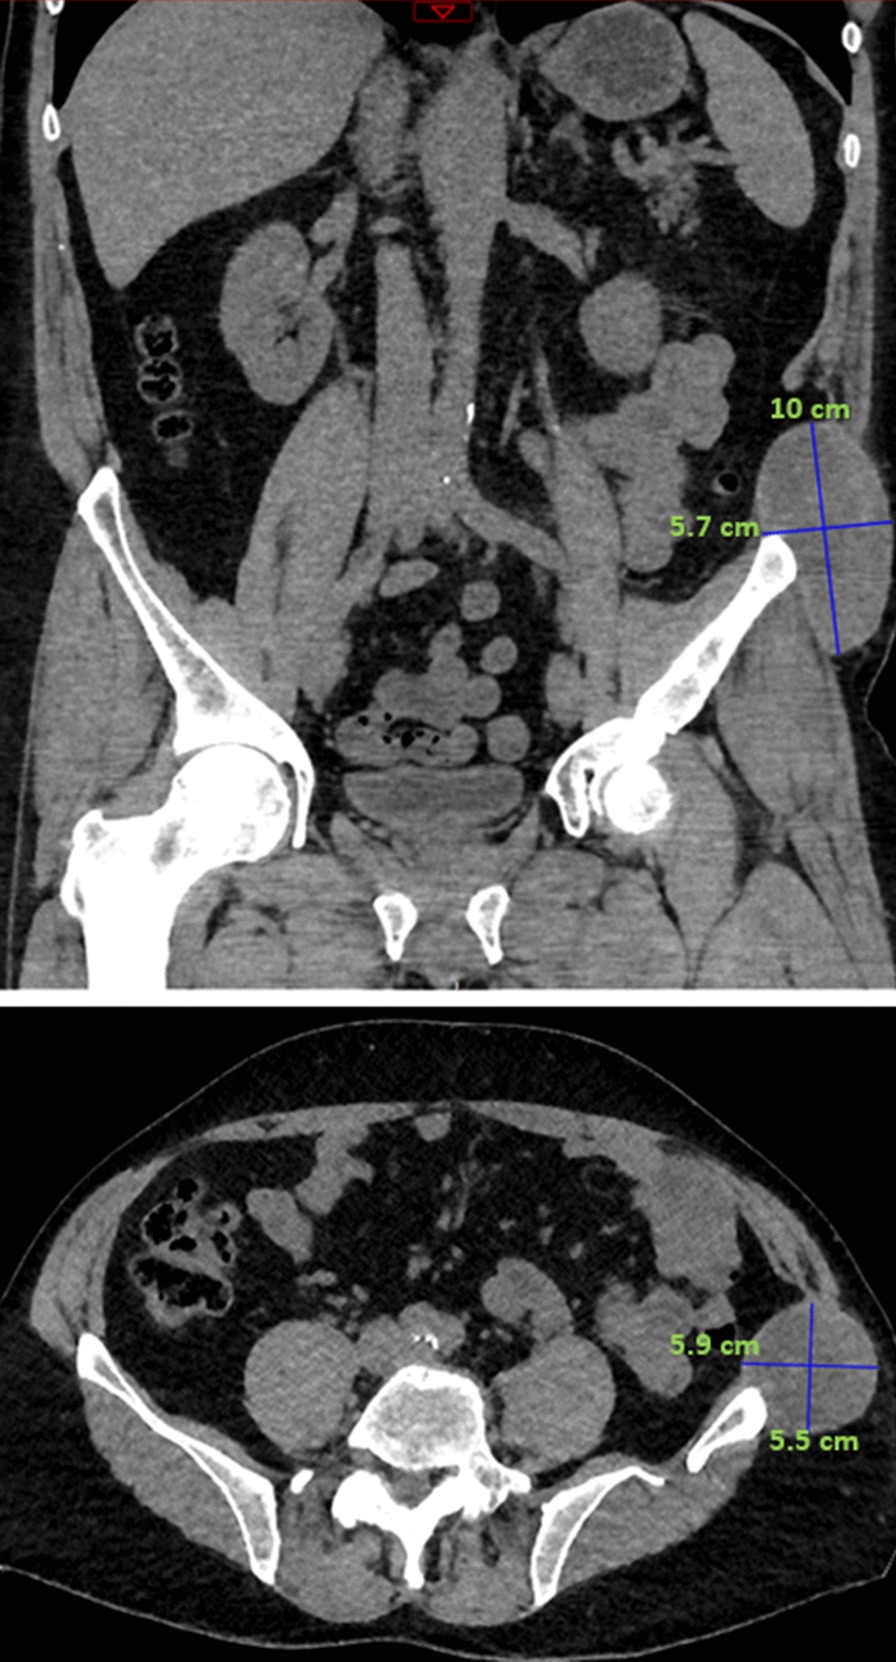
CT abdominal scan: parietal collection near the left iliac crest
